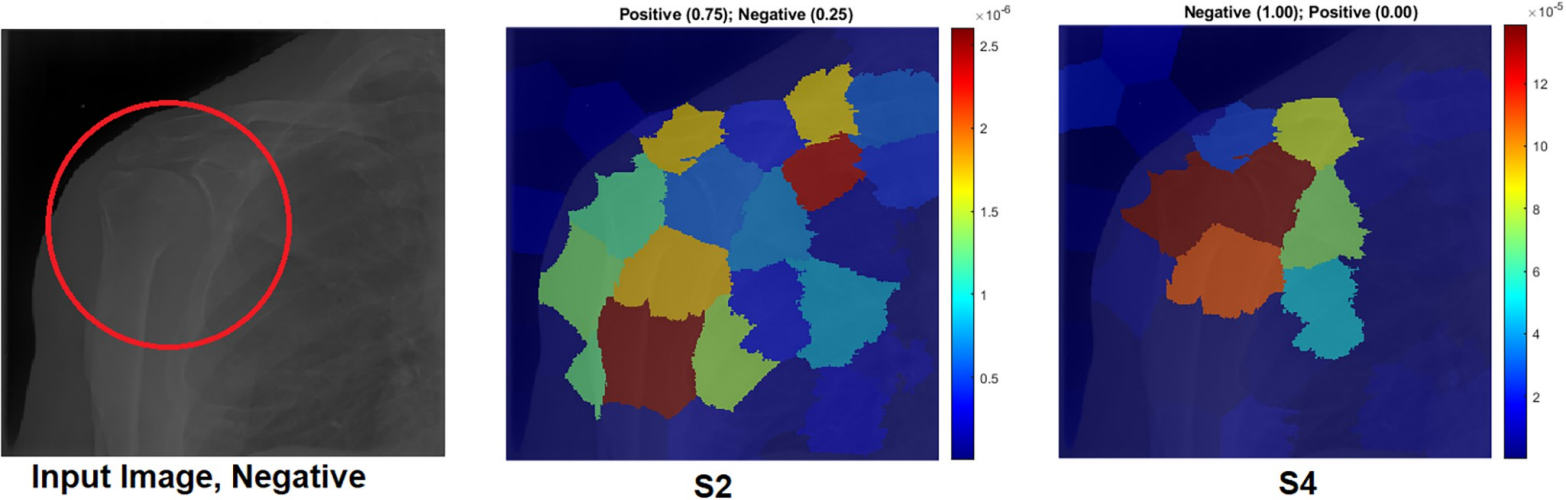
LIME and Score-LIME for shoulder X-ray image. The correct classification is Negative. The ROI is the red circle that a domain expert has marked.
